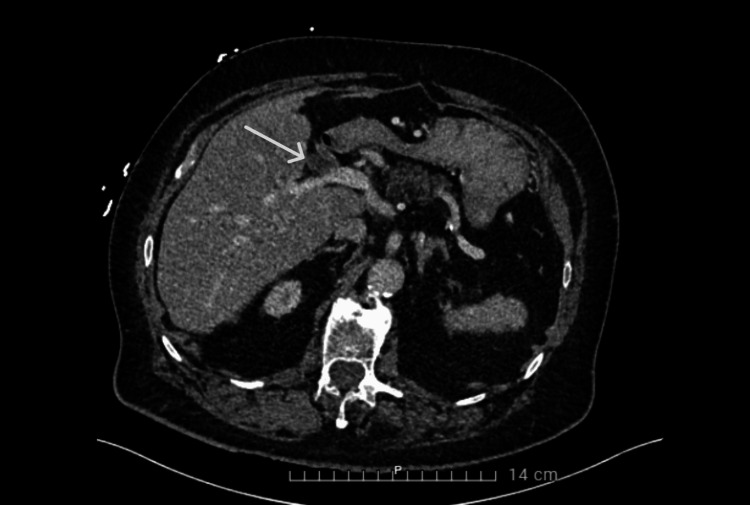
CT scan of the abdomen showing the known left portal vein thrombosis
